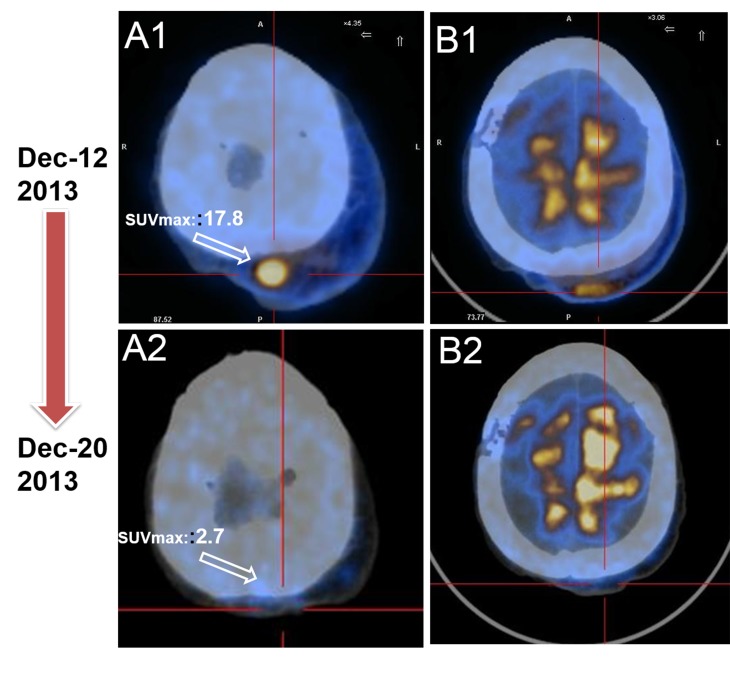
PET/CT serves as a favorable tool to evaluate the early therapy effect for EA patient A. Baseline PET/CT image before everolimust treatment revealed masses on left upper scalp, and the SUVmax was 17.8 ( December 8, 2013). B. PET/CT displayed significant decreased tumor burden with everolimus,and the SUVmax was 2.7.The case was evaluated as PR (Dec 20, 2013).
